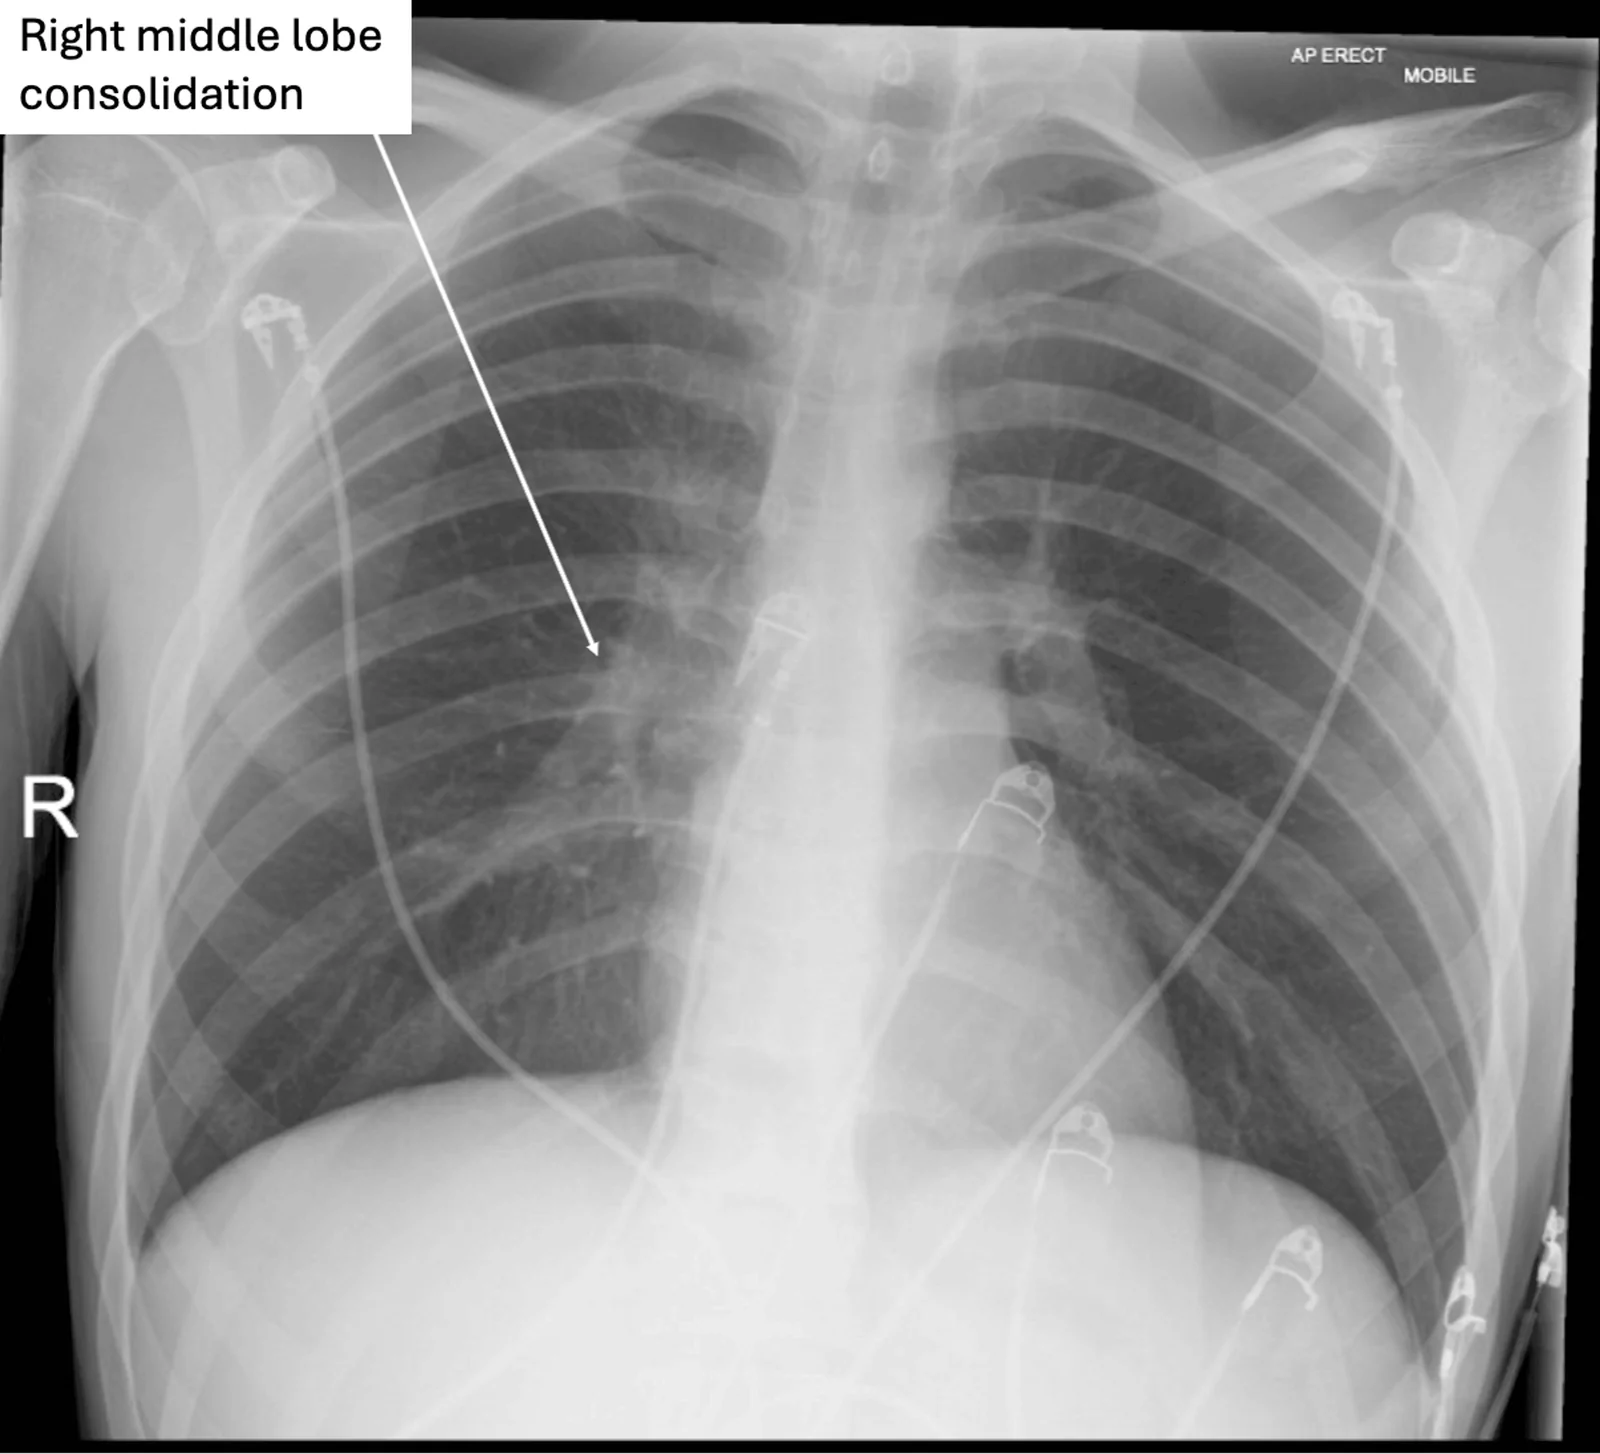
Anteroposterior chest X-ray demonstrating right middle lobe consolidation
